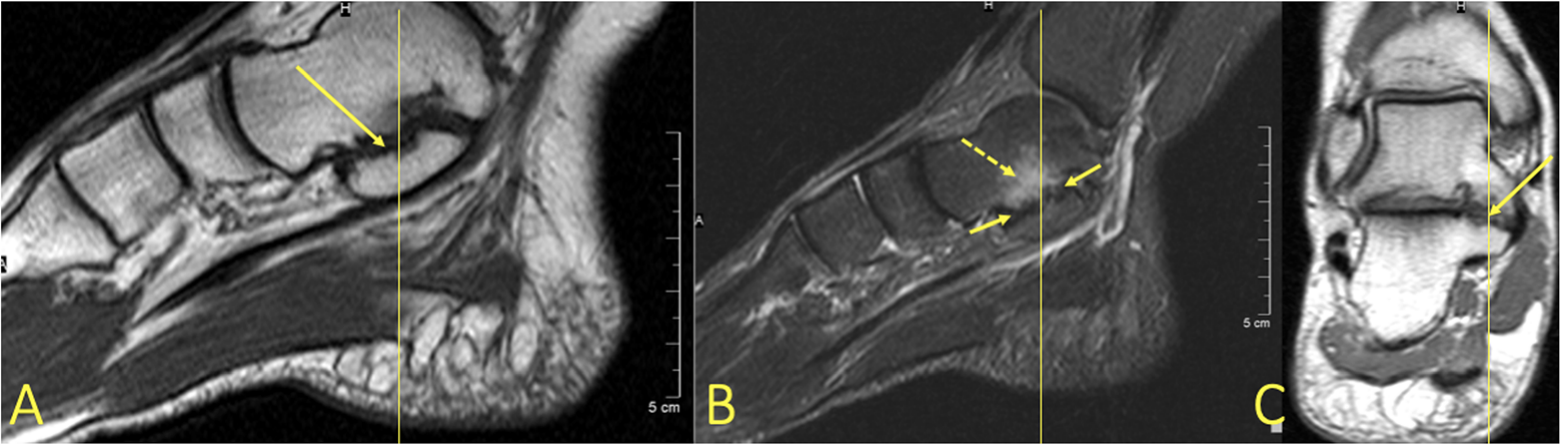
A 43-year-old female with chronic ankle pain and suspicion of an osteochondral lesion and osteoarthritis. MRI showed a talocalcaneal coalition (arrow) with BME in the talus (dashed arrow)
